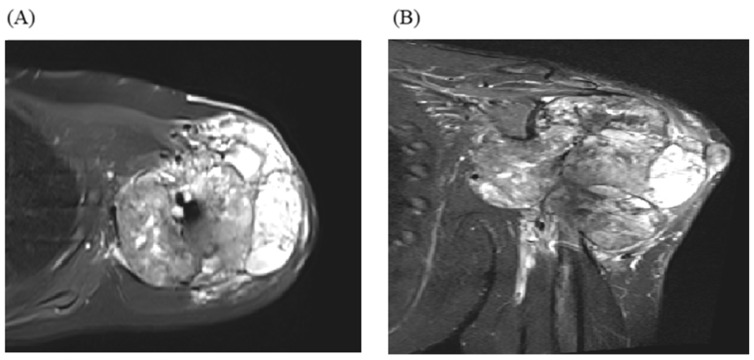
(A) Axial magnetic resonance imaging (MRI) with T2-weighted fat suppression and a coronal image (B) revealing heterogenous signal intensity with an articular extension.
